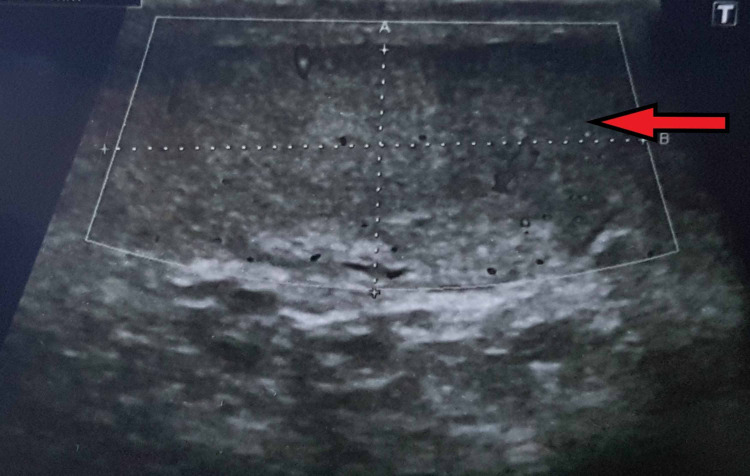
Ultrasound showing normal left testis.
